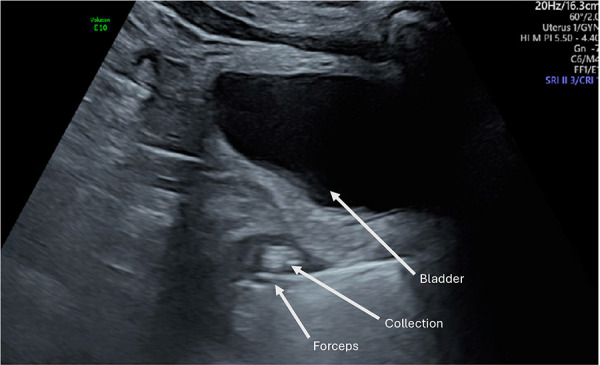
Transabdominal ultrasound image displaying forceps, infected vault collection and bladder. Sagittal view.
